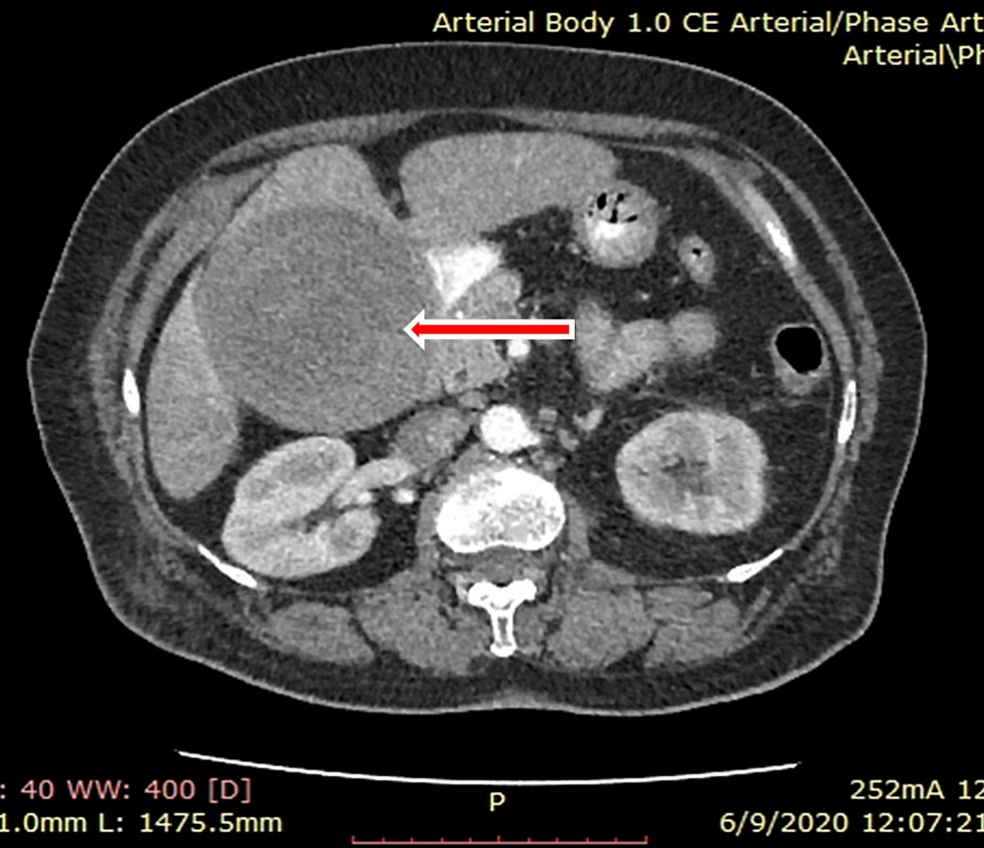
Axial cut of an abdominal CT with contrast. Heterogeneous material with a density almost equal to that of blood filling the entire distended gall bladder, with no significant contrast enhancement
